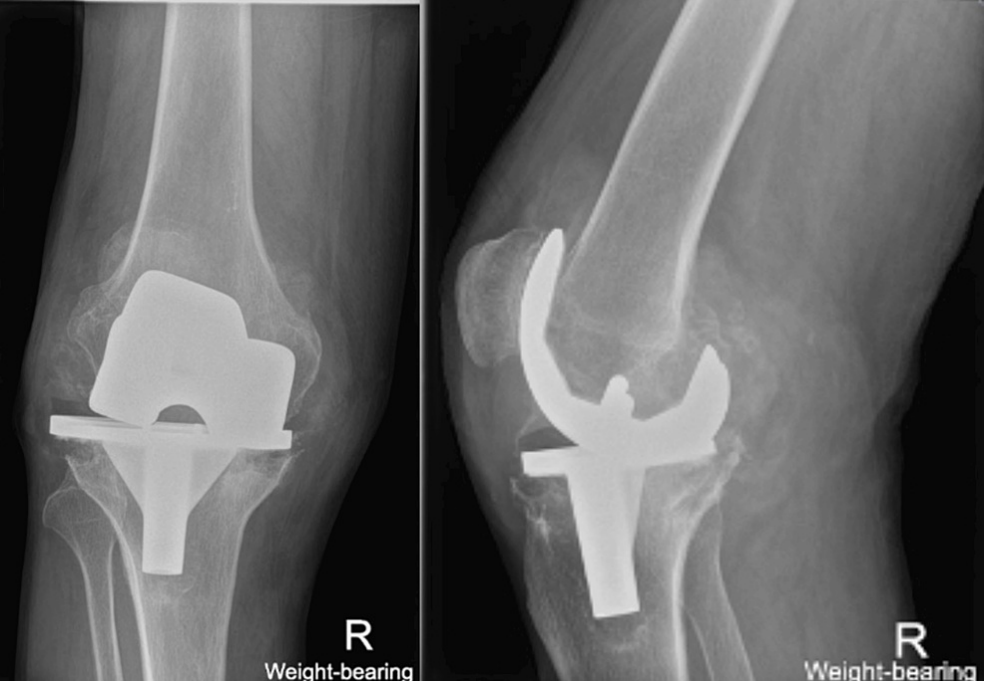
Anteroposterior and lateral X-rays of the right knee showing further wear of the polyethylene liner along with osteolysis.
